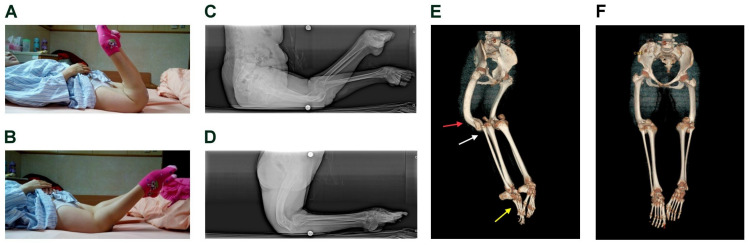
Pre-operative clinical photos and radiologic images. Photographs of the patient while lying flat on the bed show the recurvatum (hyperextension) deformity of 130° of both knees in the extension position (A) and recurvatum of 40° of both knees in the flexion position (B). The triple films show the patient using her buttock and posterior thigh to sit (C) and the popliteal fossa and calf to stand and walk (D). Pelvic to lower limb 3D-CT reconstruction (E) shows both distal femur shafts anterior bowing (red arrow) with femoral condyle hypoplasia and tibial anterior subluxation (white arrow). Additionally, both feet present as talipes equinocavovarus (yellow arrow). An anterior-posterior image of pelvic to lower limb 3D-CT reconstruction is also shown (F).
